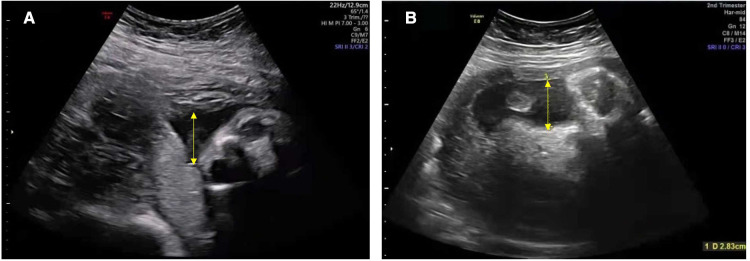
Ultrasound showing a reduced volume of amniotic fluid in the mother during pregnancy. The amniotic fluid index and the maximum depth (yellow arrow): (A) 81 mm and 33 mm at 28^+4^ weeks; (B) 47 mm and 28 mm at 34^+5^ weeks (reference ranges for the index of amniotic fluid and the maximum depth: >80 mm and >30 mm), respectively.
